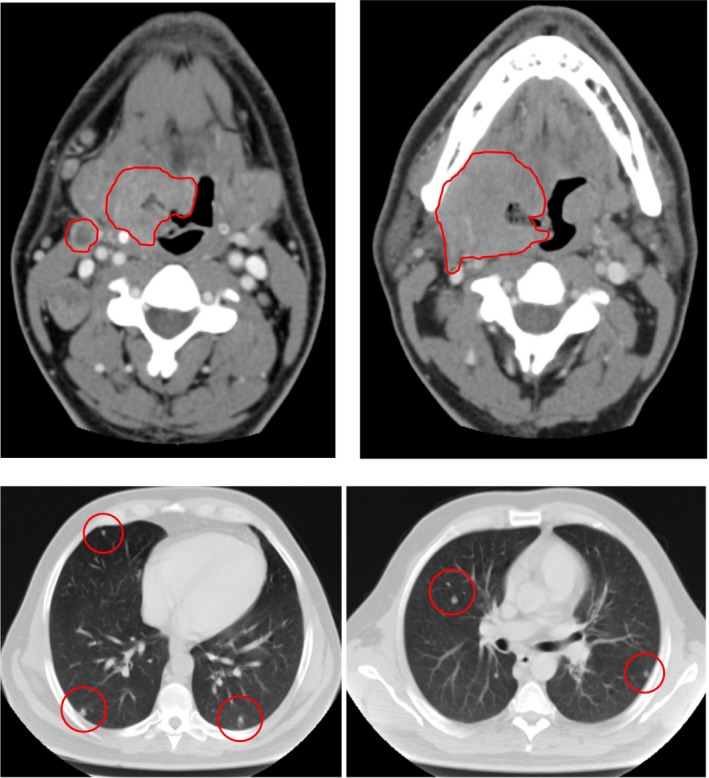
June 2013; first staging with computed tomography.
